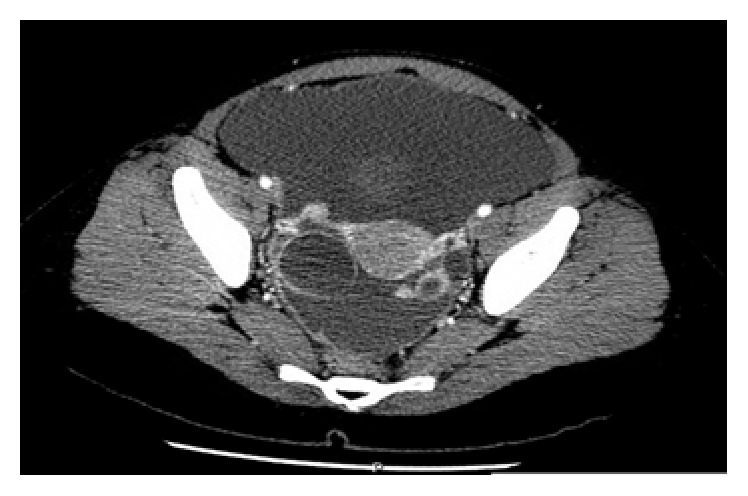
CT scan showing rim enhancing cystic lesions in the right and left adnexa measuring 3.5 cm and 1.4 cm, respectively, with multiple other nodules in both adnexa, peritoneal thickening, and abdominopelvic ascites.
